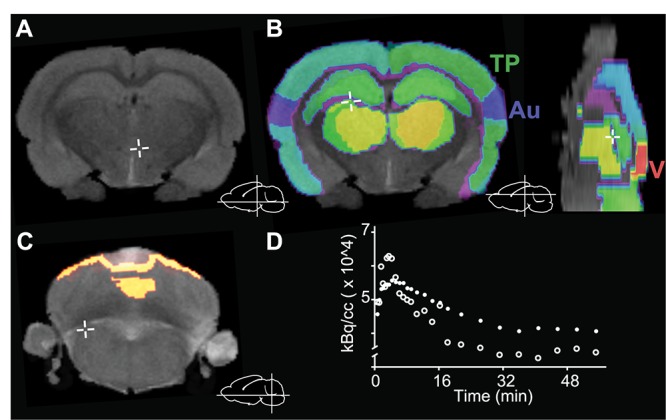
Image processing and anatomical structure segmentation. (A) 7T *in vivo* MR images were obtained and (B) used for manual segmentation into nine regions of interest (ROIs). (C) The cerebellum was used as reference region for data analysis. (D) Representative time activity curve of the reference region (cerebellum, open circles) and the rest of the brain (all ROIs except cerebellum, filled circles). Au, auditory cortex; TP, temporoparietal cortex; V, visual cortex. Insets (A–C) depict the position of the cursor (cross) for each panel.
